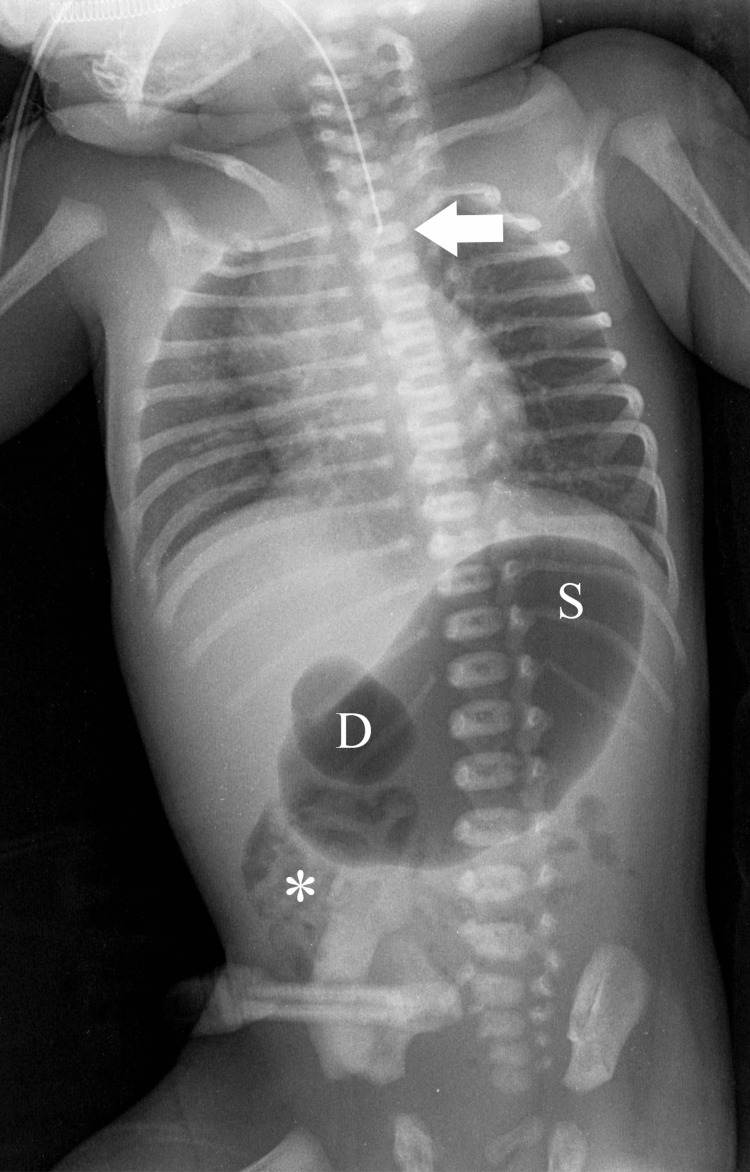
Plain X-ray of the thorax and abdomen indicating esophageal atresia and showing a “double bubble” sign The distal tip of the nasogastric tube (arrow) is superimposed in the midline at the level of the first thoracic vertebra, while distal air in the abdomen (asterisk) is noted, consistent with esophageal atresia with tracheoesophageal fistula. Marked dilation of the stomach and duodenum, namely the “double bubble sign”, is identified. D, Duodenum; S, Stomach
